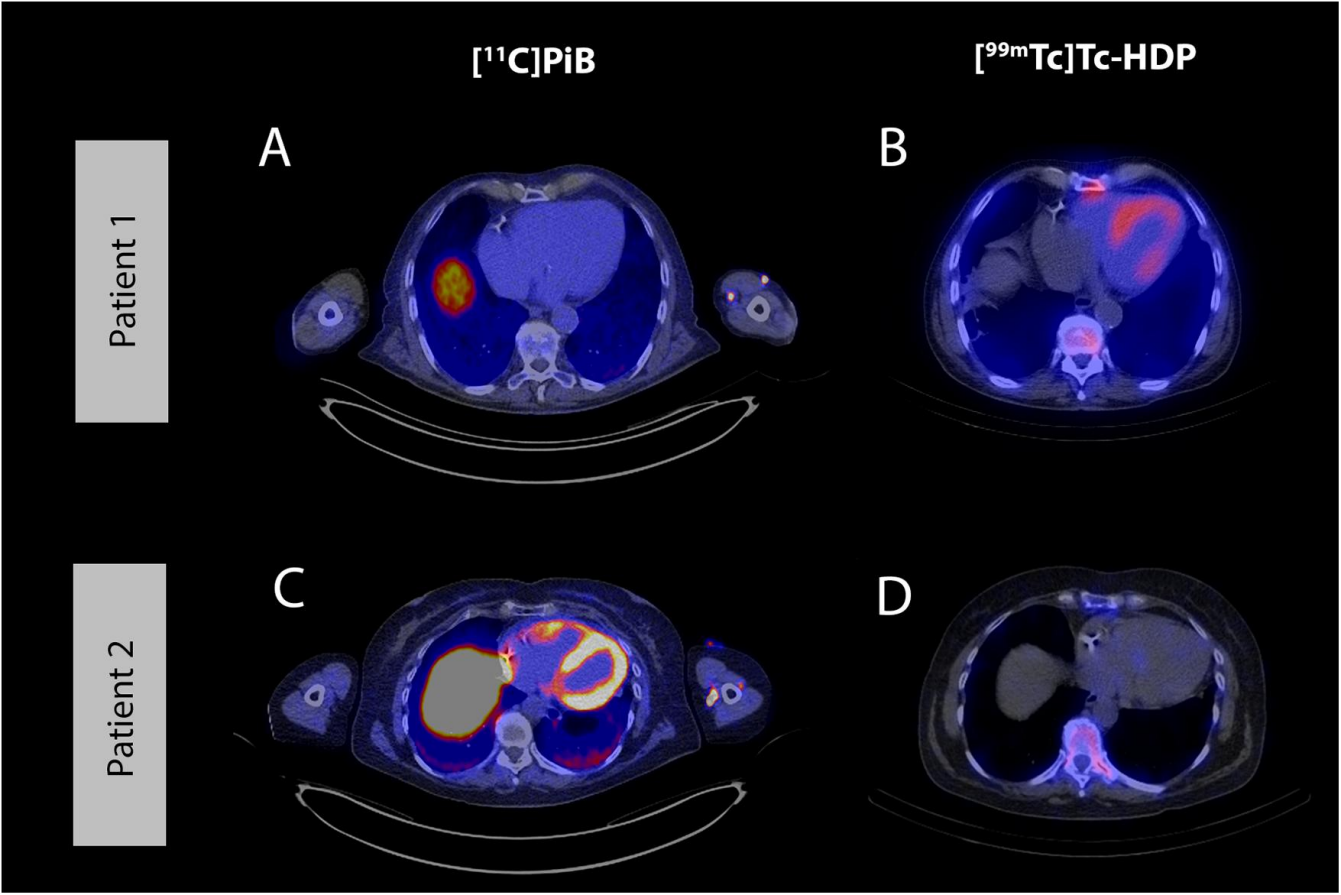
Example cases. Example of a negative [^11^C]PiB PET scan and positive [^99m^Tc]Tc-HDP SPECT/CT scan in an ATTRwt amyloidosis patient (A and B) and an example of a positive [^11^C]PiB PET scan and negative [^99m^Tc]Tc-HDP SPECT/CT scan in an ATTRv amyloidosis patient, carrying the early p.(Val50Met) variant (C and D). Axial [^11^C]PiB PET/CT images (A and C) and axial [^99m^Tc]Tc-HDP SPECT/CT images (B and D). [^11^C]PiB, Pittsburgh Compound B; PET, positron emission tomography; CT, computed tomography; [^99m^Tc]Tc-HDP, hydroxydiphosphonate; SPECT, single photon emission computed tomography; ATTRv, hereditary transthyretin amyloidosis.
